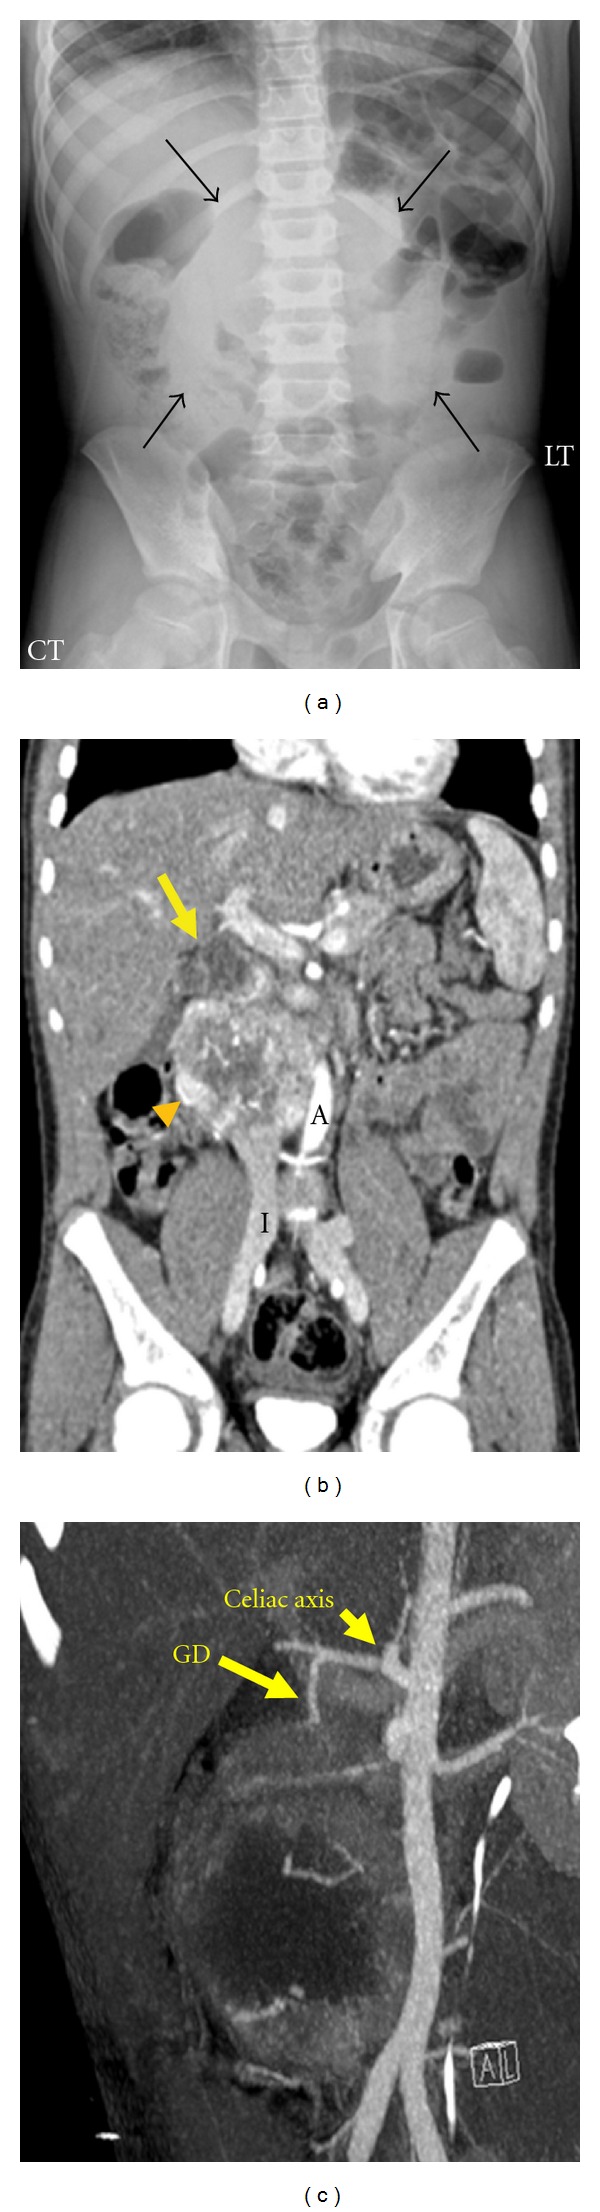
(a) Abdominal radiograph shows midline displacement of bowel loops by a soft tissue density (black arrows) without calcifications and no bowel obstruction. (b) Coronal CTA shows the markedly enhancing mass (orange arrowhead) displacing the aorta (A) and IVC (I) and its close proximity to the 3rd portion of the duodenum (yellow arrow). (c) A maximum intensity projection from the CTA depicts gastroduodenal artery GD (arrow) as the primary feeder of the paraganglioma.
